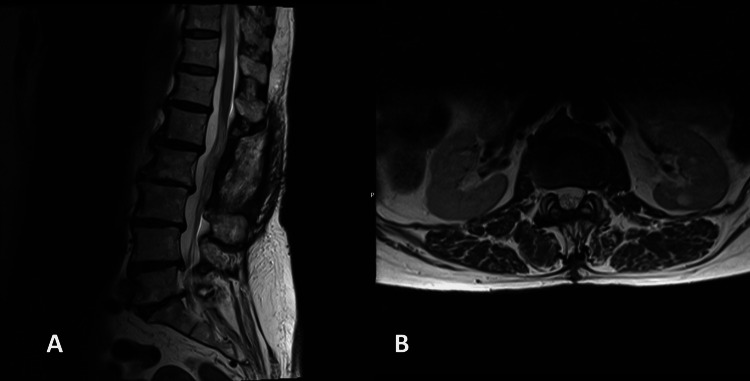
Postoperative MRI showing no recurrence (A) Sagittal T2 and (B) axial T2
